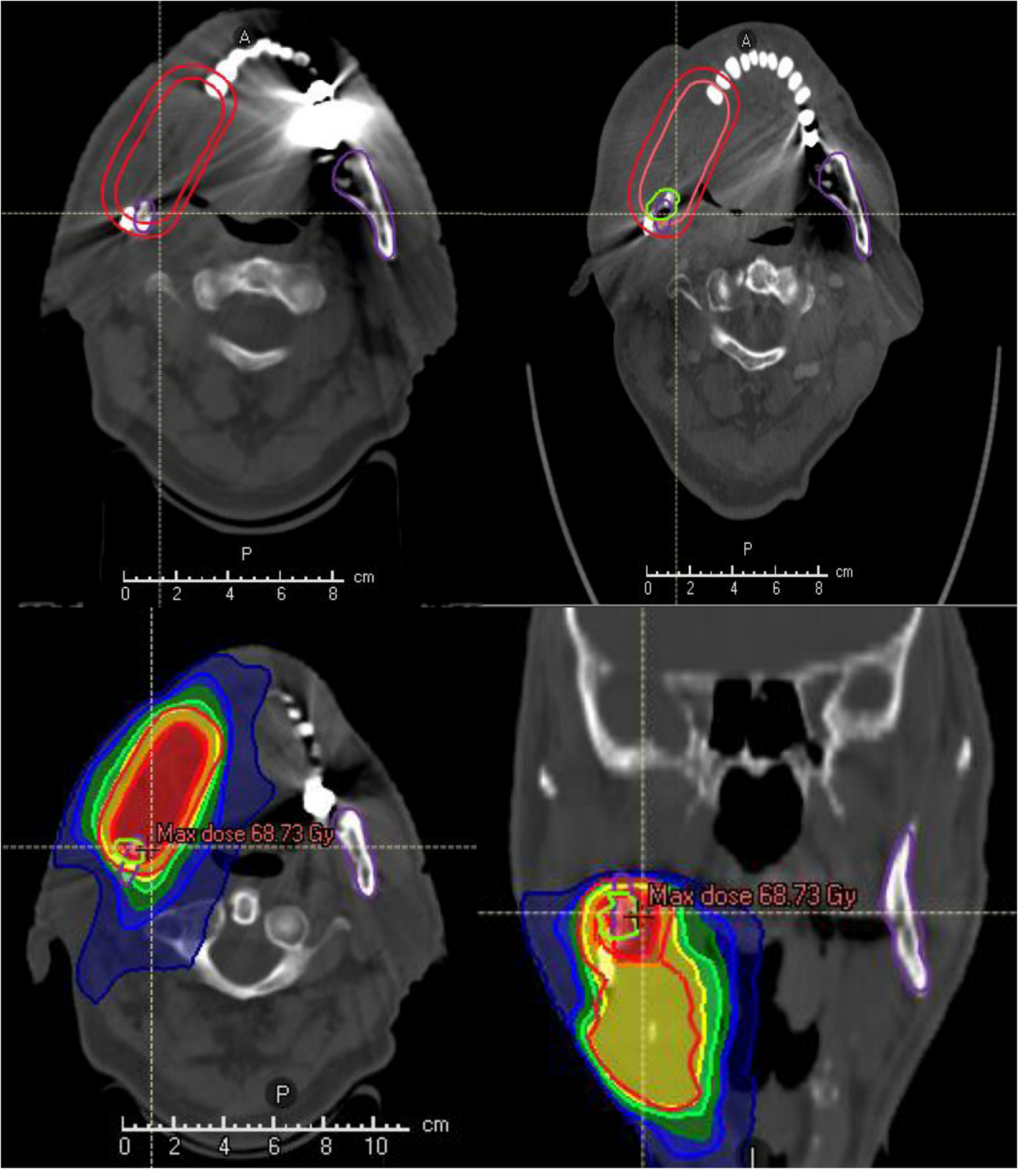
The images a–d show the planned CT in one patient with oral cavity cancer undergoing postoperative radiotherapy, including PTV that was planned to receive a total dose of 66.0 Gy (single dose 2.0 Gy) in 33 fractions. To the top right image b shows the ORN of the lower jaw (colored in green) in the high-dose region with maximum dose 68.7 Gy. Therefore, we matched the follow-up CT with the planned CT and dose distribution, and then volumetric and dosimetric evaluations were performed
